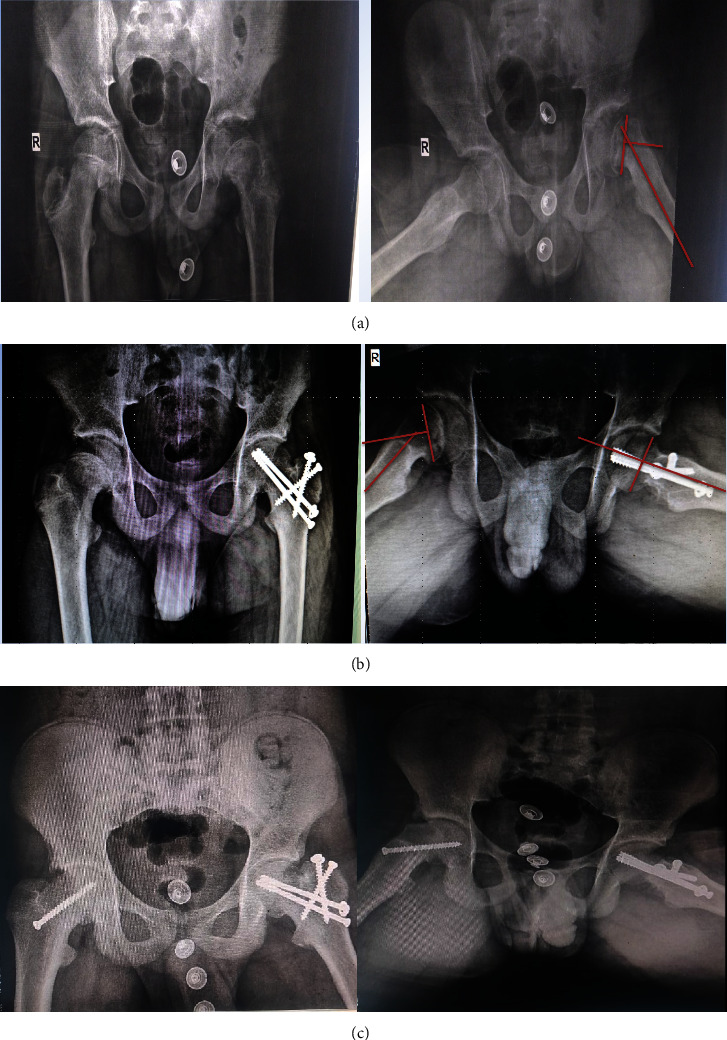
AP and frog lateral radiographs of a 14-year-old male patient with left stable chronic moderate SCFE. (a) Preoperative. (b) 1 year postoperative showing anatomical reduction and complete union of osteotomies and incidental finding of contralateral mild SCFE. (c) Pinning in situ of the right side and development of FAI which is listed for arthroscopic debridement.
